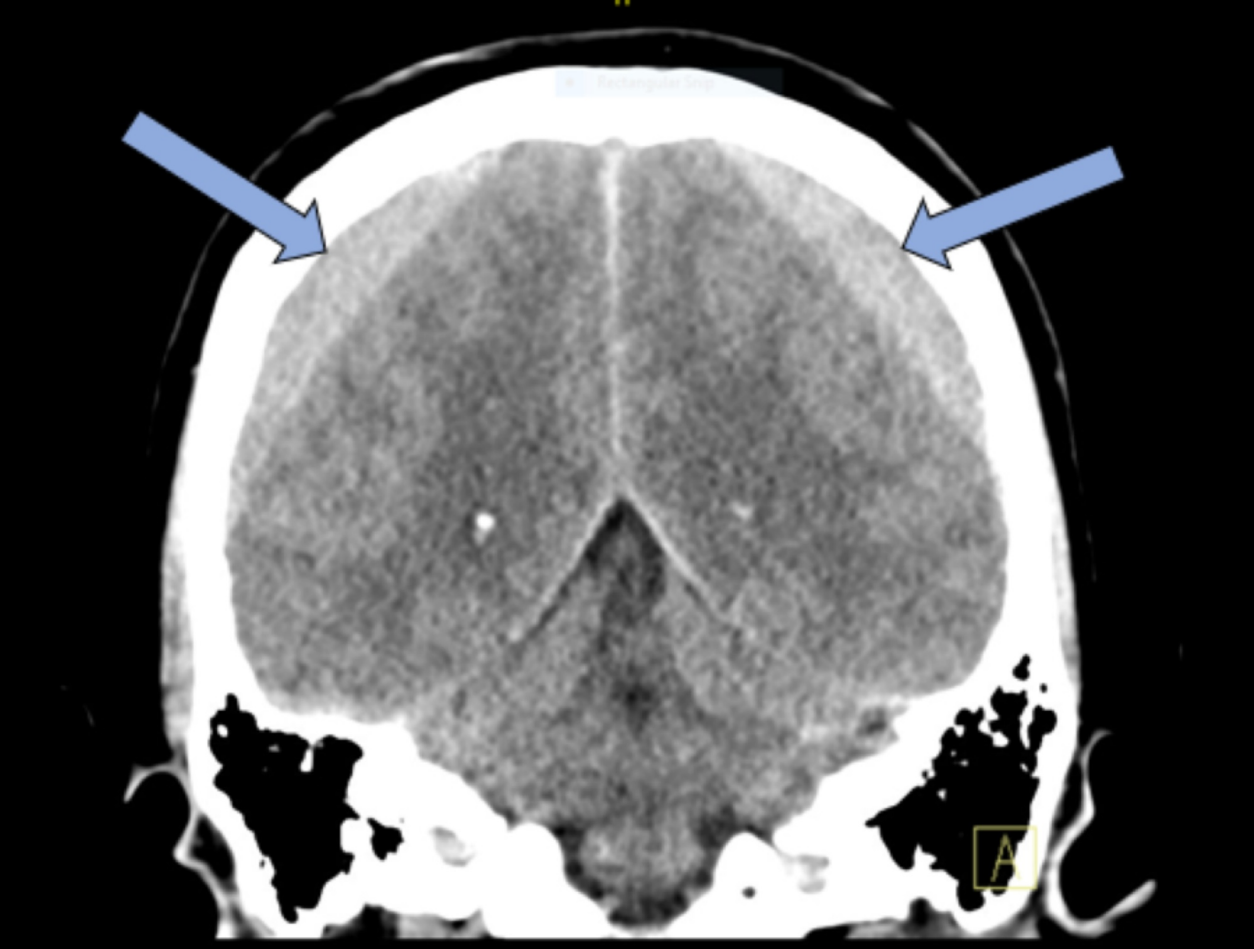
Coronal slice of a non-contrast head computed tomography demonstrating bilateral symmetric subacute subdural hematomas (blue arrows) with sulci effacement.
